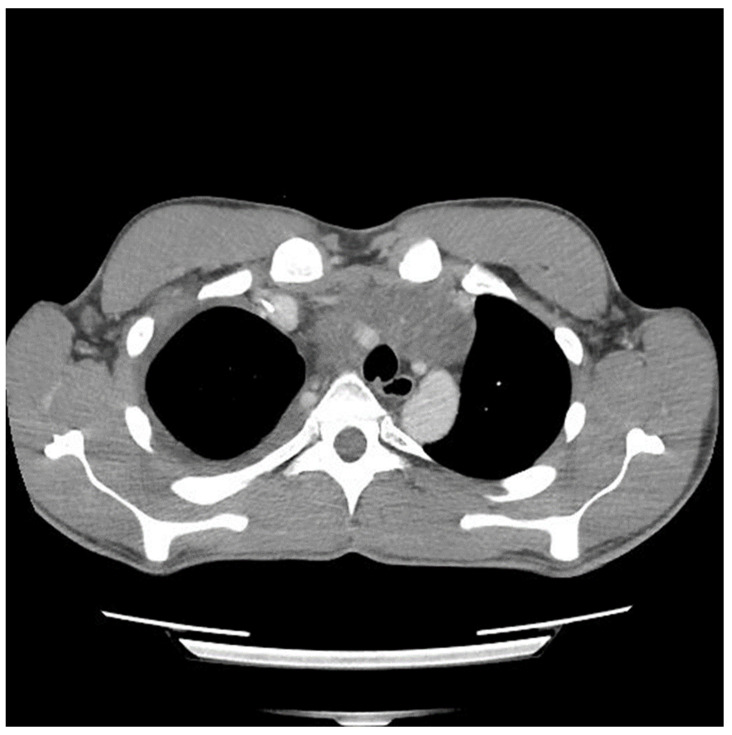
Computed tomography (CT) scan image of mediastinal mass after two cycles of chemotherapy showing encasement of left subclavian artery.
